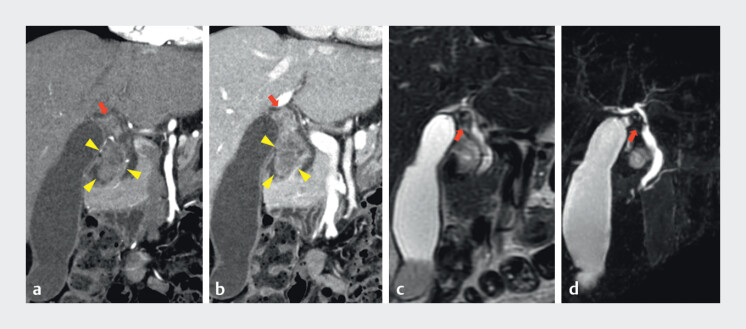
Computed tomography image and magnetic resonance cholangiopancreatography.
